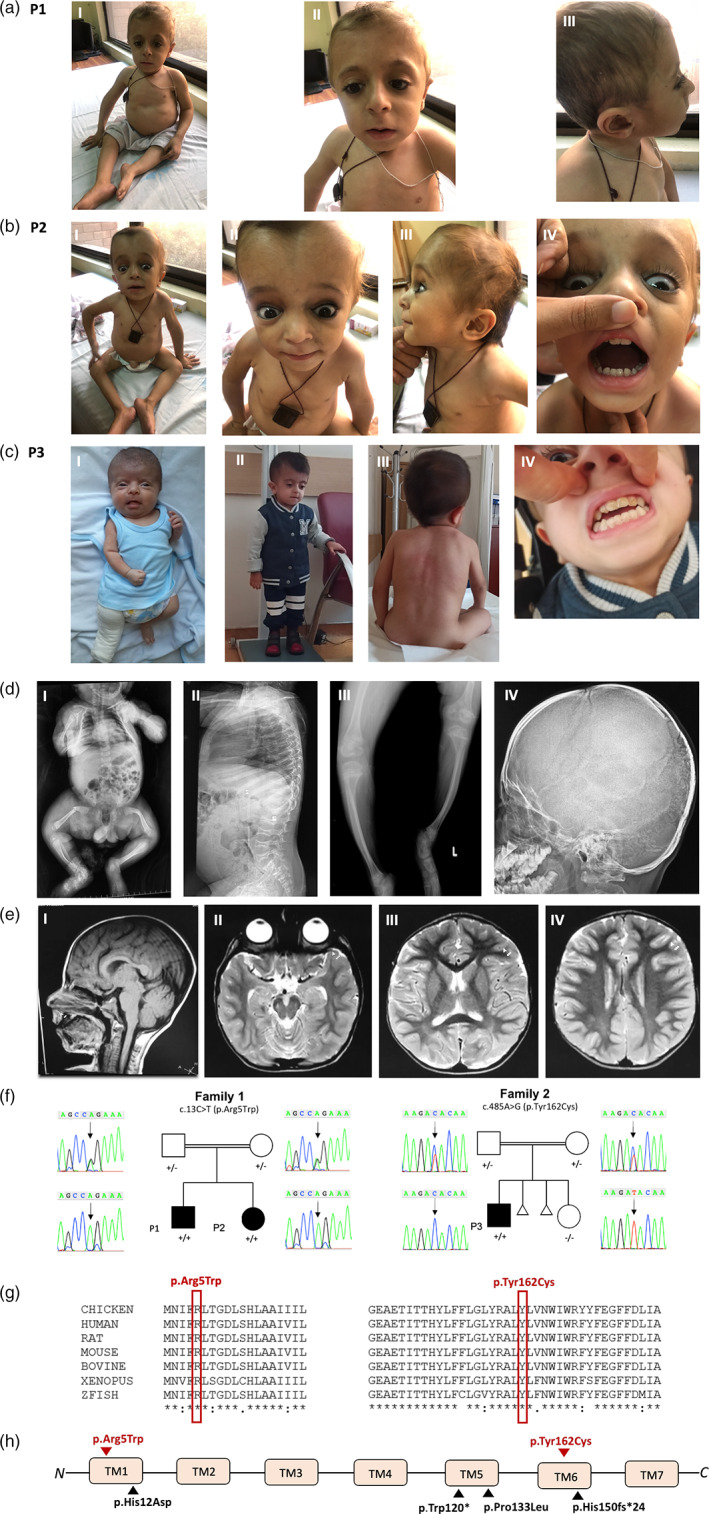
Three affected patients with *KDELR2*‐related osteogenesis imperfecta from two consanguineous families. (a) Photographs of patient P1 showing short stature, barrel shaped chest (I), sunken eyes, epicanthus inversus (II), and sparse thin hair (III). (b) Photographs of P2 showing short stature, barrel shaped chest (I), blue sclera (II), sunken eyes secondary to molding of the soft cranium (II), thin sparse hair (III), and dentinogenesis imperfecta (IV). (c) Photographs of P3 showing infantile short stature a right leg cast following a pathological femoral fracture (I), current short stature at age 4 years (II), scoliosis (III), and dentinogenesis imperfecta (IV). (d) Radiographs of affected subjects depicting infantile femoral fracture from P3 (I), vertebral compression fractures and platyspondyly from patient P1 (II), short bowed limbs from P1 (III), and Wormian bones from P1 (IV). (e) Brain MRI sections from P1 obtained at 6 years of age. (I) Sagittal T1 showing normal brain appearance. (II) Axial T2 showing brachycephaly. (III and IV) Axial T2 images showing age‐appropriate myelination. (f) Sanger segregation of *KDELR2* variants in family 1 and 2. (g) Conservation of amino acid residues across species for both variants. (h) Location of current (red) and previously reported (black) *KDELR2* pathogenic variants. All identified variants to date affect transmembrane domains (TMs) 1, 5, and 6 of the KDELR2 protein product [Color figure can be viewed at wileyonlinelibrary.com]
